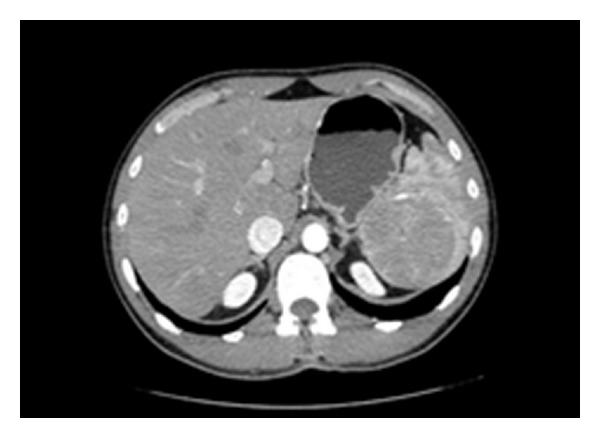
Computed tomography showing a hypodense mass of the spleen with contrast enhancement during arterial phase.
